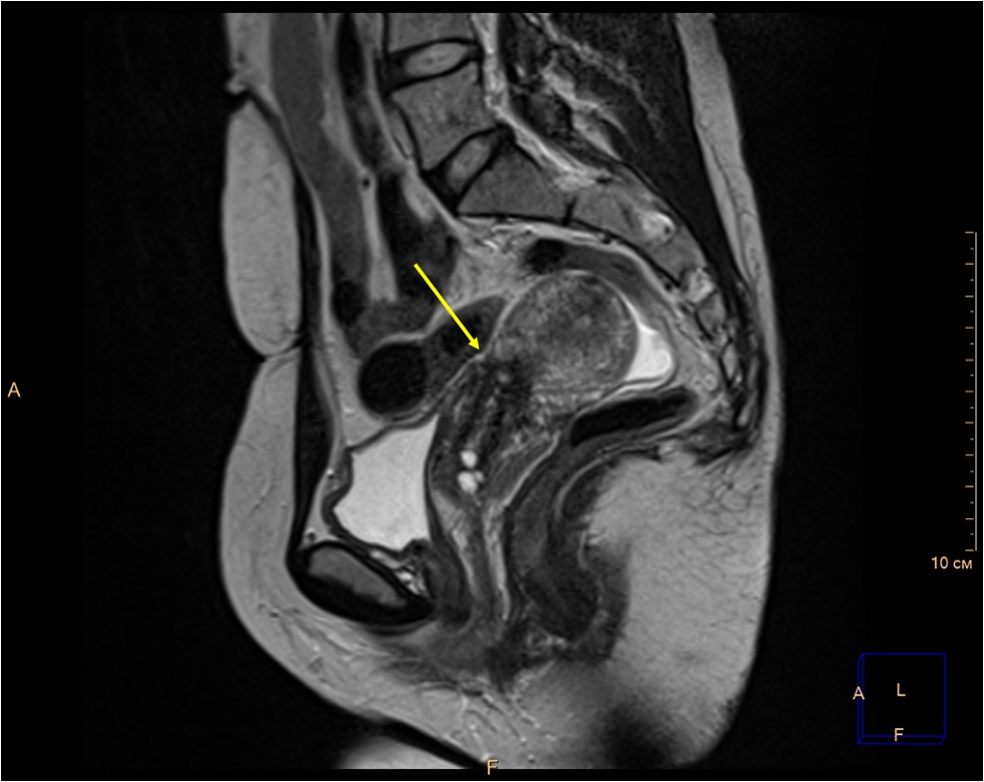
Pelvic MRI, T2-weighted sagittal plane. The *yellow arrow* indicates the cesarean section scar. No signs of a vesicouterine fistula are present
